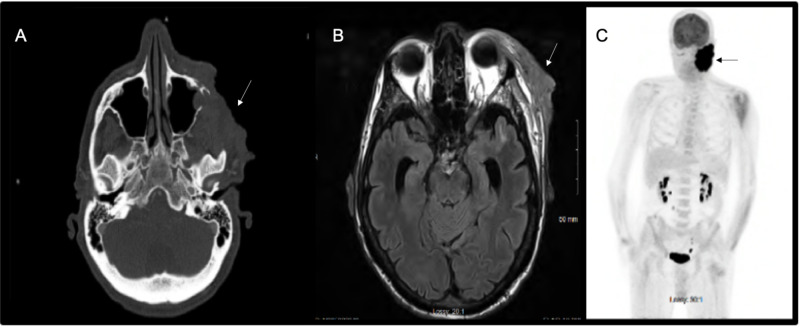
(A) Brain CT scan; (B) Brain MRI; (C) PET scan Radiographic workup of the lesion demonstrates (A) CT axial 8.9-cm mass with the invasion of the soft tissues of the left face, with involvement and bony destruction of the left zygomatic arch and the lateral wall of the left maxillary sinus; (B) MRI T2-FLAIR axial image demonstrating a mass in the left frontozygomatic region invading the lateral orbital region extraconal; (C) whole-body coronal PET scan demonstrating increased FDG-uptake in the left facial neoplasm CT, computed tomography; MRI, magnetic resonance imaging; PET, positron emission tomography
